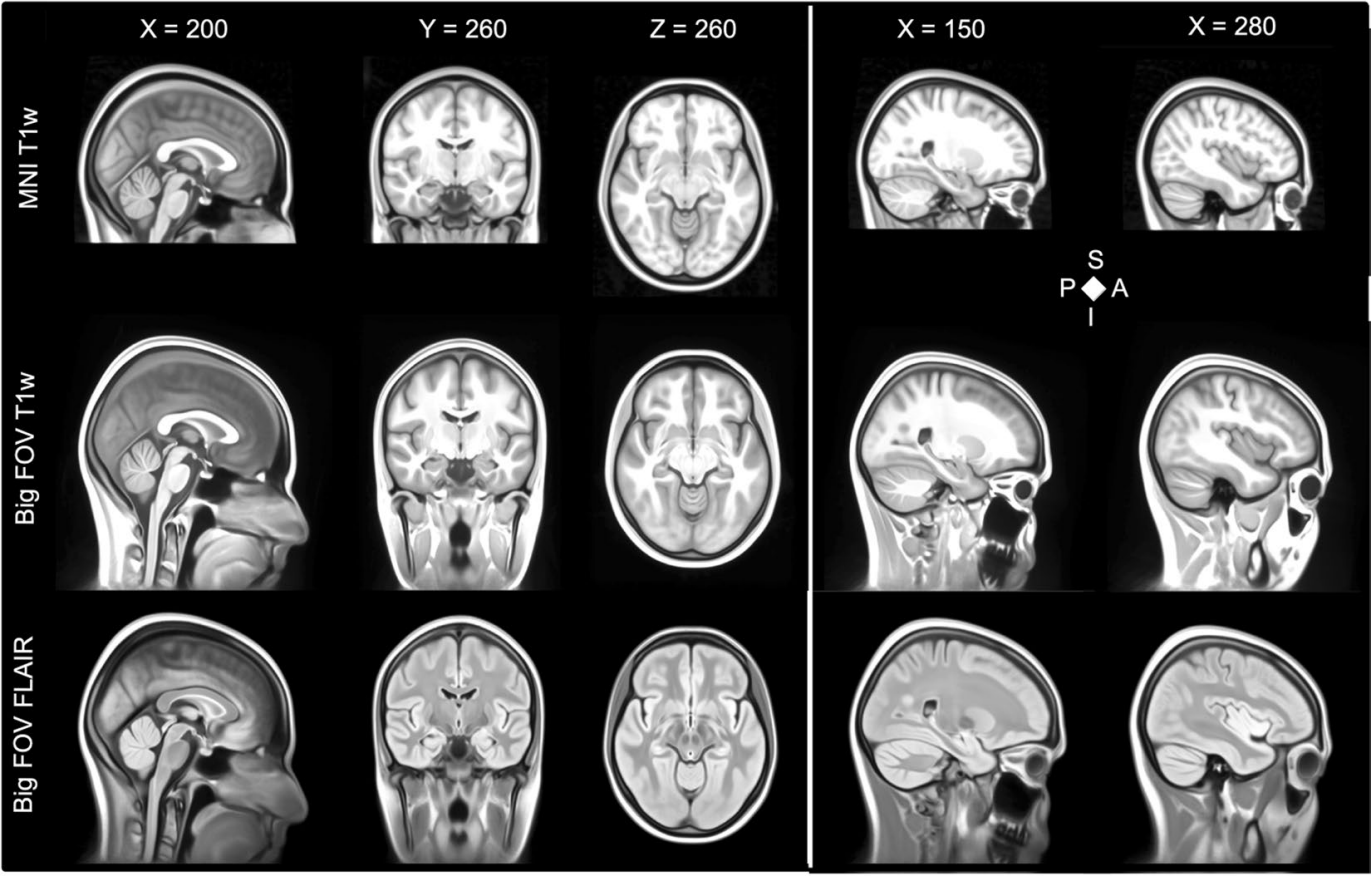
Generated full FOV templates of T1w and FLAIR in 0.5 mm^3^ resolution in comparison to the MNI T1w template. S = superior, P = posterior, I = inferior, A = anterior, T1w = T1-weighted image, MNI = Montreal Neurological Institute, FOV = field-of-view, FLAIR = fluid-attenuated inversion recovery. Images are in radiological convention (left in the image is right in the subject/template).
